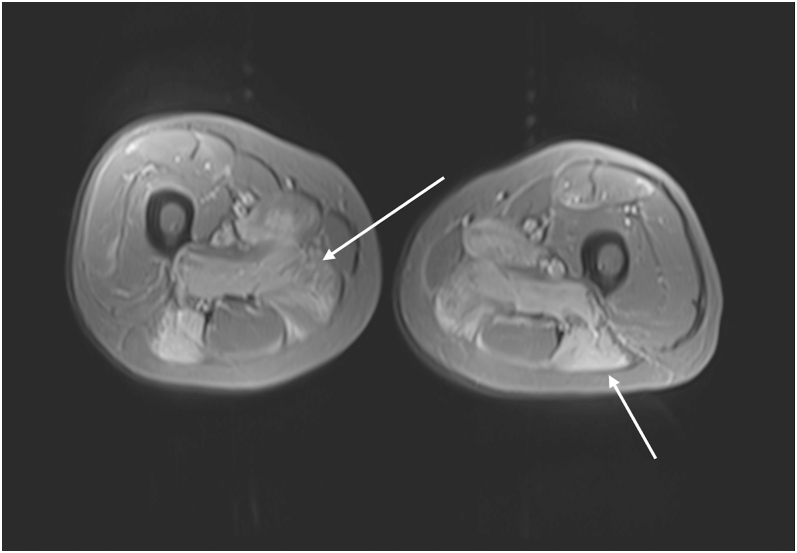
Axial short tau inversion recovery (STIR) MRI images of the lower limb. High signal intensity in the posterior and medial compartments of the thigh, in keeping with myositis. Imaging limited by motion artefact.
